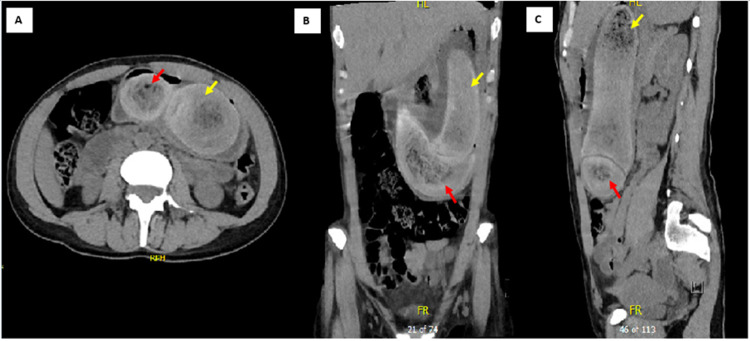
Axial (A), coronal (B) and sagittal (C) computed tomography imaging of the abdomen and pelvis showed two well-defined foci in the distended stomach. The larger focus (yellow arrow) is seen within the gastric lumen while the second focus (red arrow) extends distally into the pyloric antrum
